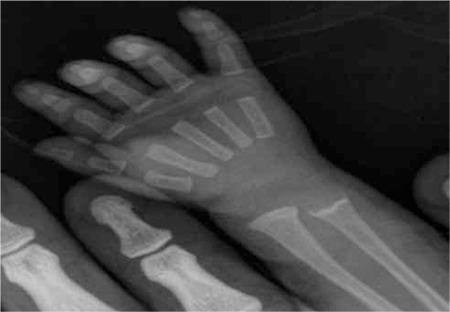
Case 1- wrist X-ray demonstrating metaphyseal cupping and irregularity
